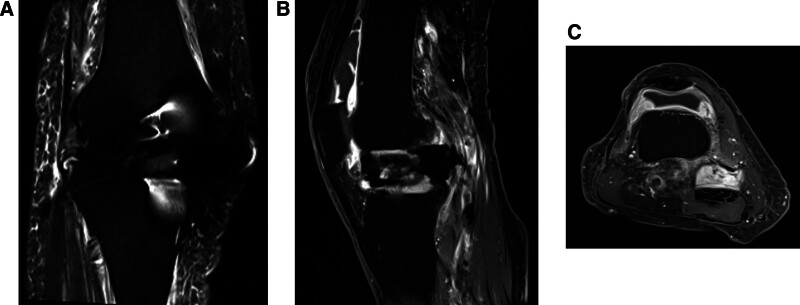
(A) The coronal view of T2-fat suppression MRI for the right knee joint. (B) The sagittal view of T1-weighted enhanced MRI for the right knee joint. (C) The axial view of T1-weighted enhanced MRI for the right knee joint.
